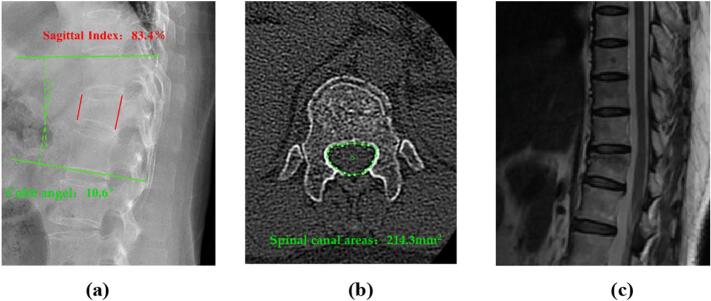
Lateral X-ray, transverse CT and sagittal MRI were performed 3 months before admission.
